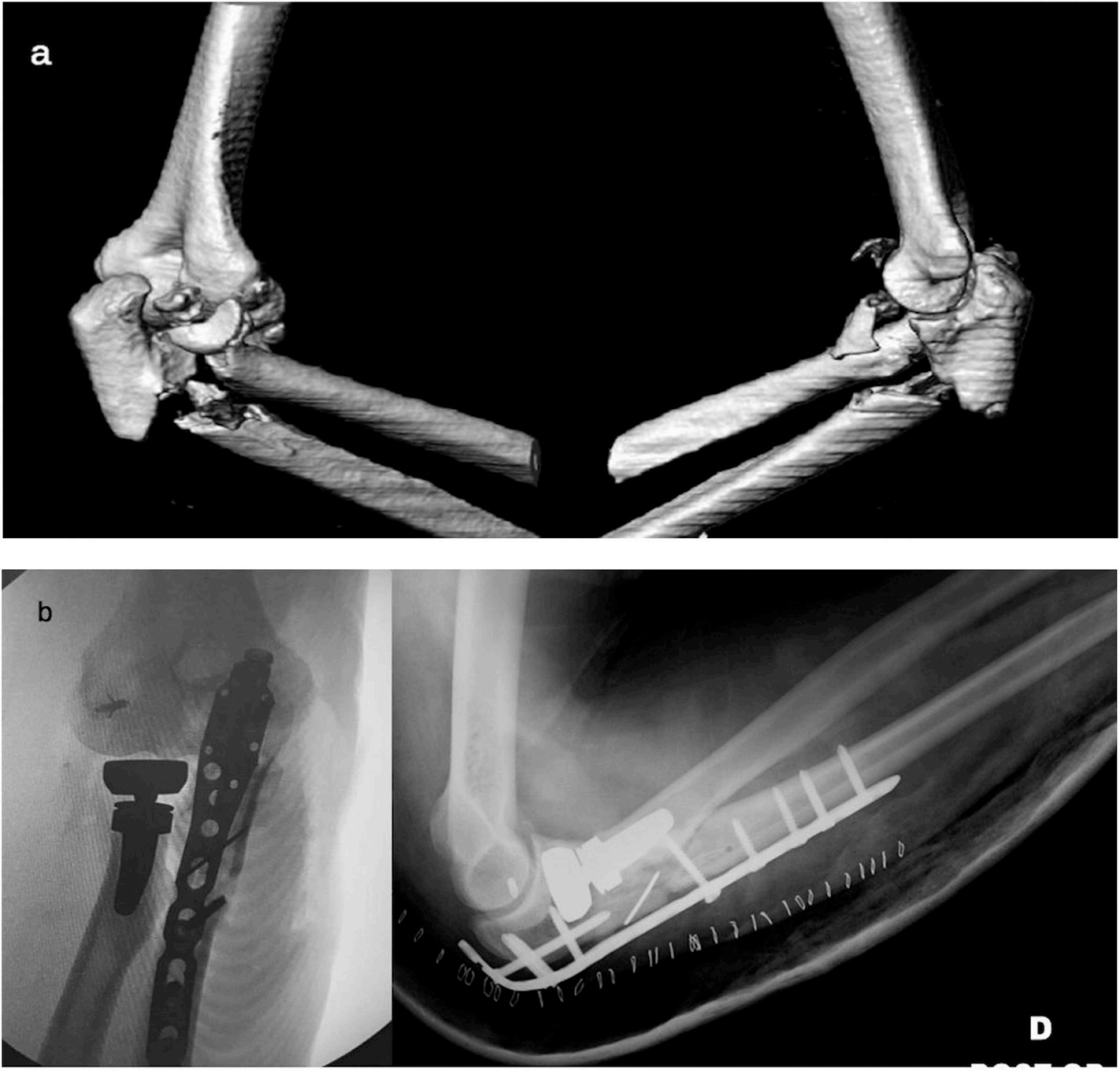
a) 3D-CT scan: comminuted fracture of the proximal ulna and of the radial head in a 58-year old man. b) Post-operative radiography: global posterior approach and subcutaneous Kocher approach – Pre-contoured locking compression plate, cemented radial head prosthesis and suture anchor fixation of lateral ulnar collateral ligament.
